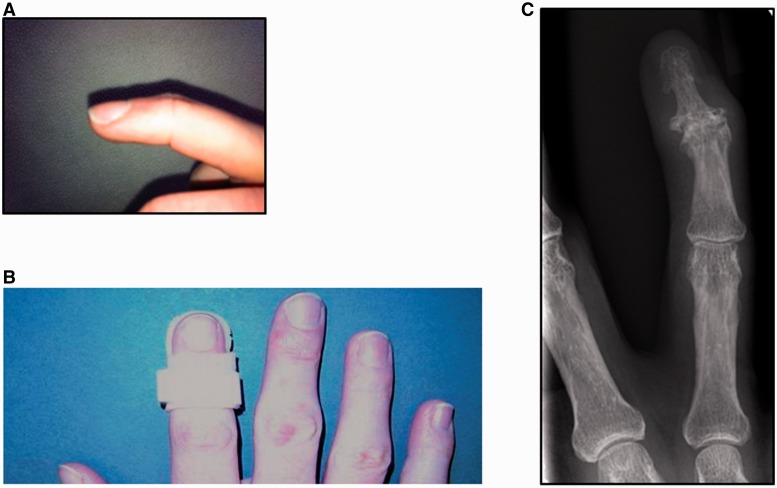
Custom thermoplastic gutter splinting of the DIP joint (A) An example of an extension lag deformity of the distal IP joint prior to splinting. There is incomplete extension at the joint on attempted active extension by the individual. (B) Splints were fabricated from thermoplastic by a senior hand therapist and adjusted at 6 weeks if necessary to ensure comfort and fit. An example is shown. (C) Anteroposterior plain radiograph of a digit from a study subject showing an affected middle finger DIP joint on the right hand. Evidence of radiographic change consistent with OA of the joint is present and there is also radial deviation deformity.
